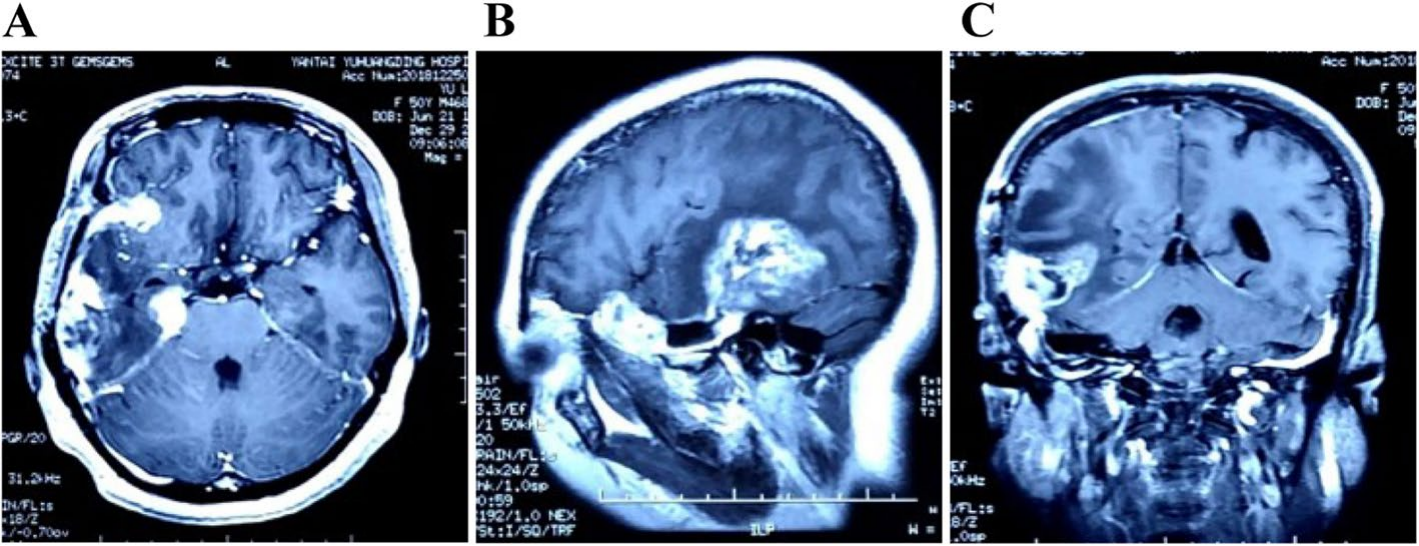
After the second admission, the preoperative MRI scan showed the recurrent tumor in the right temporal lobe. Multiple enhanced nodes had been spread to frontal lobe and extended to subtemporal area. A Enhanced axial scan. B Enhanced sagittal scan. C Enhanced coronal scan
